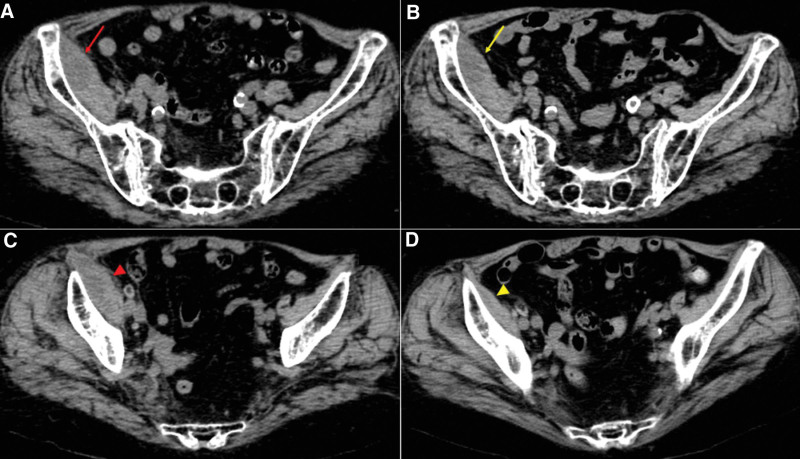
(A and B) Computed tomography (CT) on admission revealed a solitary right iliac fossa abscess sized 1.9 cm × 6.0 cm × 5.2 cm (red arrow and arrowhead) (A, sacroiliac level; B, lower sacral level). (C and D) A follow-up CT on day 16 showed a shrinking abscess (yellow arrow and arrowhead) (A, sacroiliac level; B, lower sacral level).
